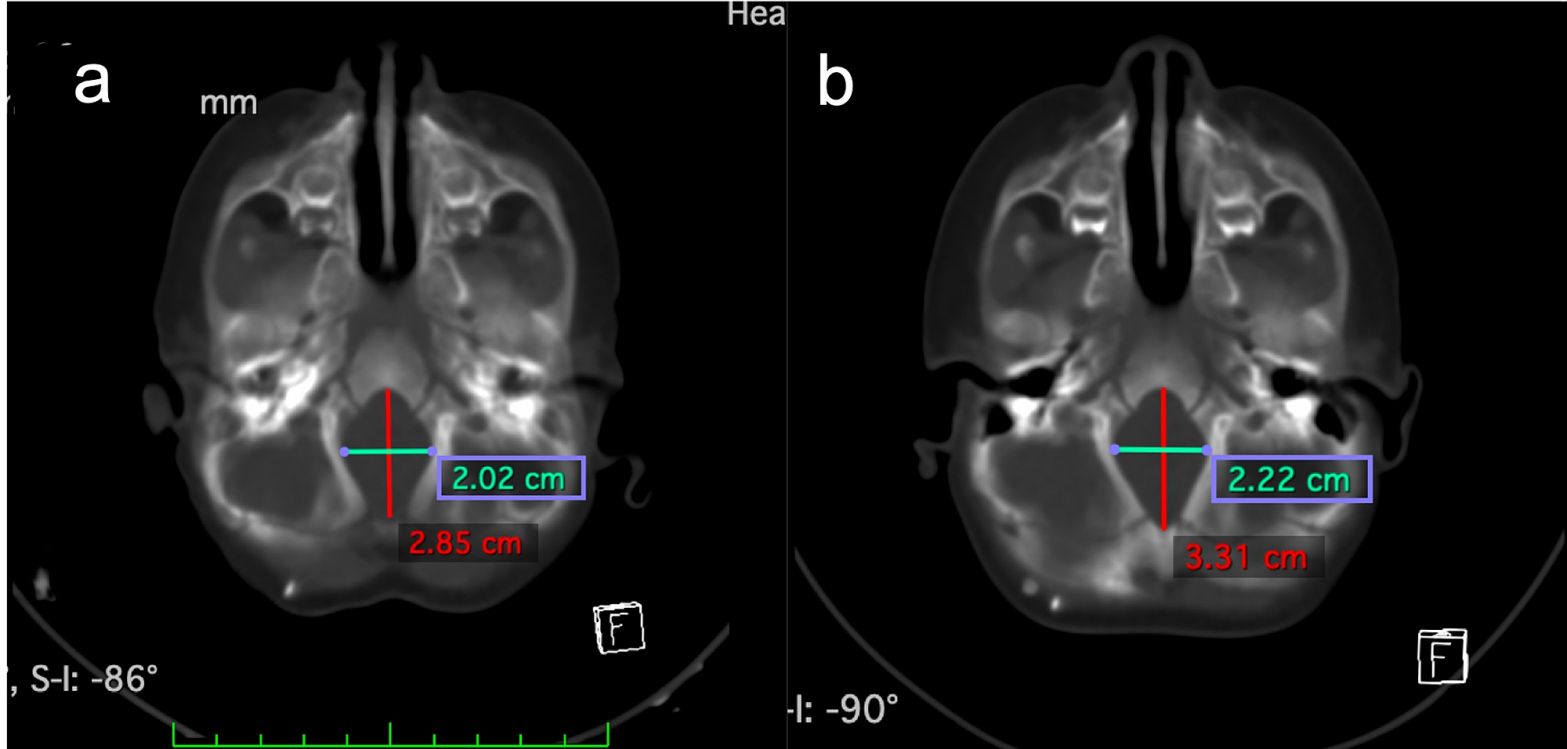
Foramen magnum Pre-operative (a) and post-distraction (b) axial CT dimensions of the foramen magnum.
